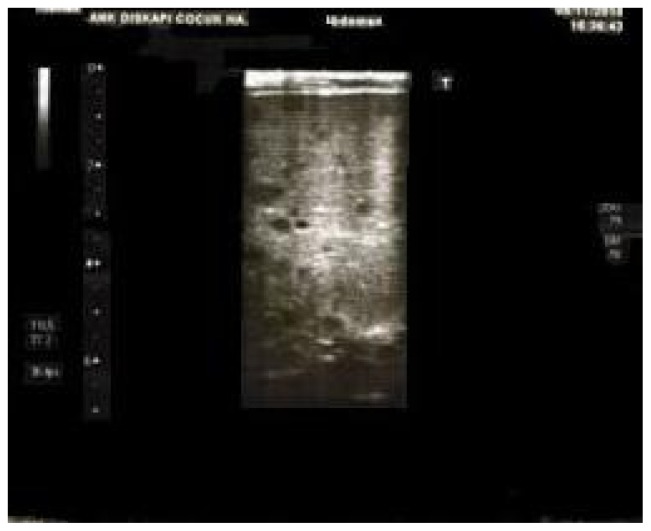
The sonographic appearance of multiple hypoechoic millimetric hepatic nodules.
